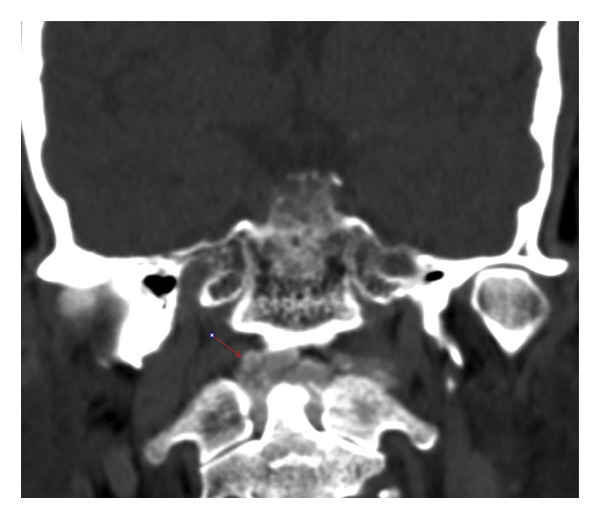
Coronal noncontrast CT of the craniocervical junction demonstrating curvilinear calcium mineralization surrounding the odontoid process, consistent with periodontoid calcification at the C1‐C2 level (arrow).
